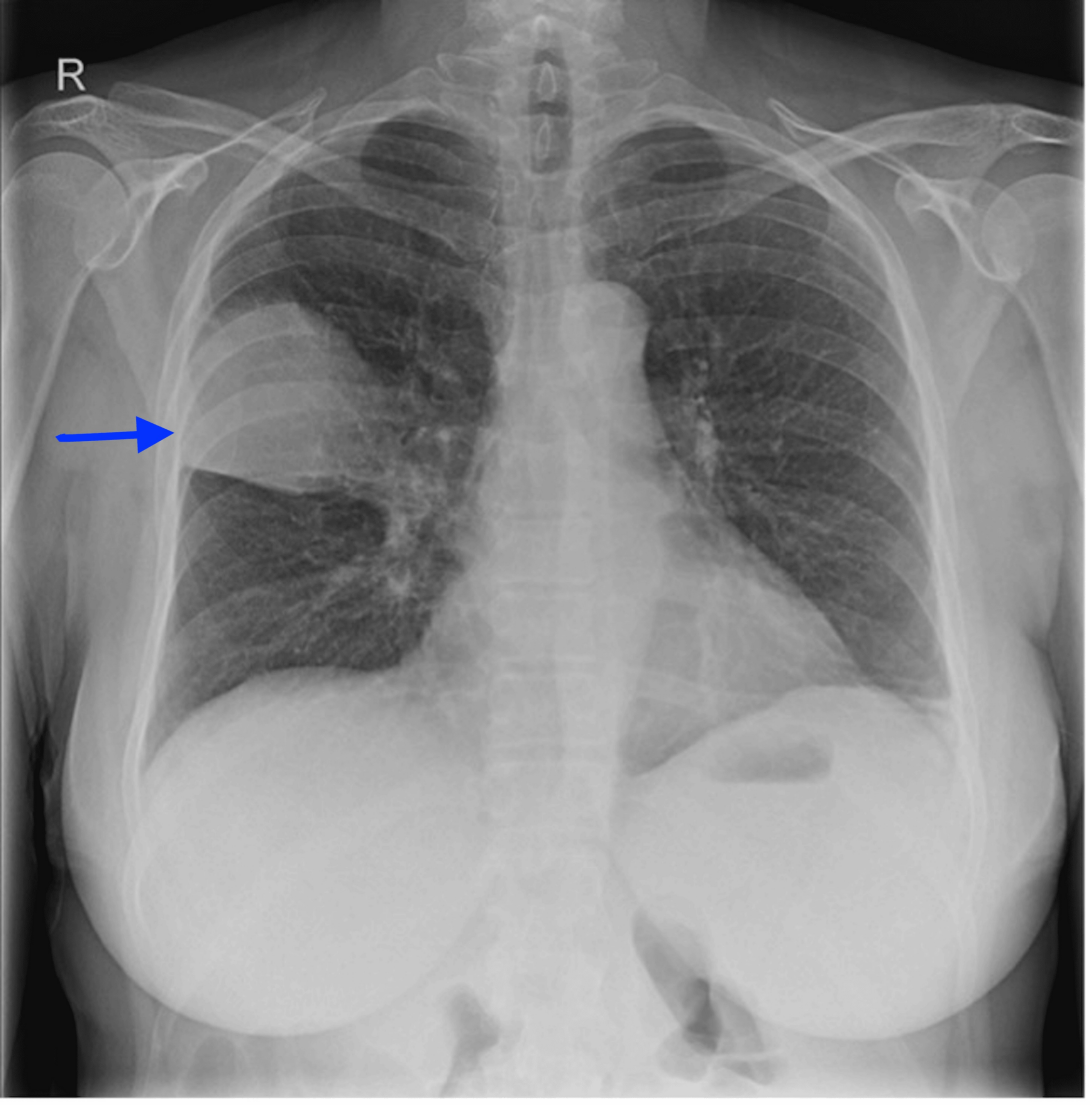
X-ray at presentation shows a consolidation in the right upper lobe
